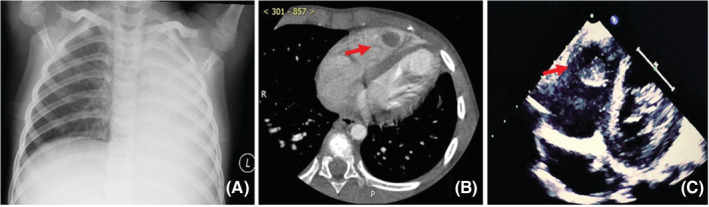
(A) Chest X‐ray at admission showed consolidation of the left lung; (B) the enhanced computed tomography taken after admission indicated a filling defect in the RV; (C) echocardiography was taken after admission, which showed a mass attached to the interventricular septum in the RV.
